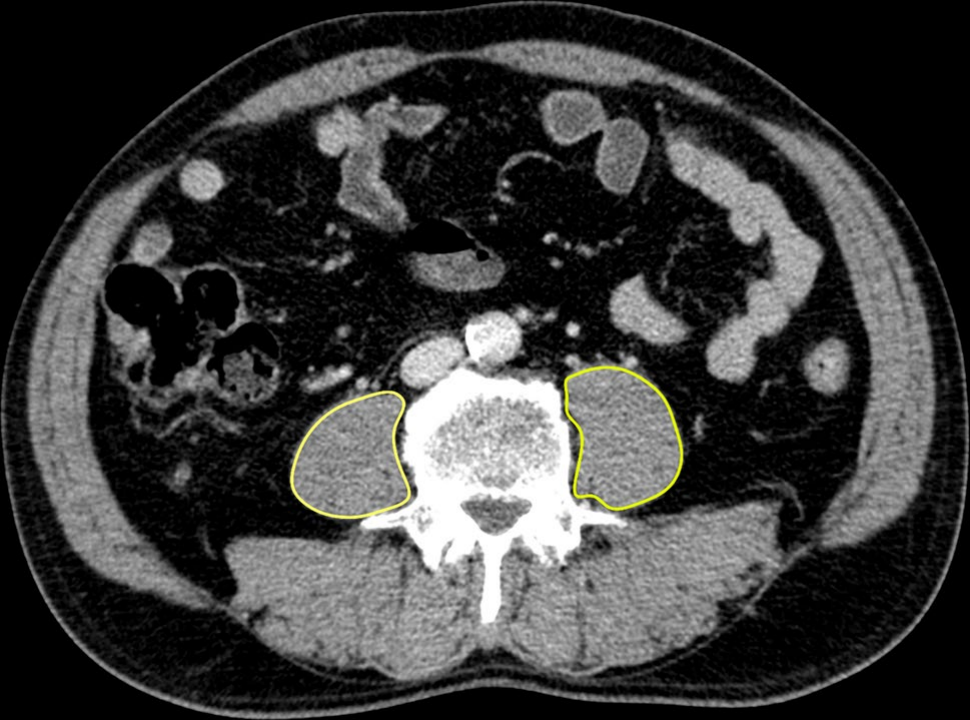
Psoas muscle area (PMA) measurement on a preoperative CT scan cross-section, calculated at the level of the 3rd lumbar vertebra. Psoas muscle margins are manually delineated to obtain both surface areas (yellow lines)
